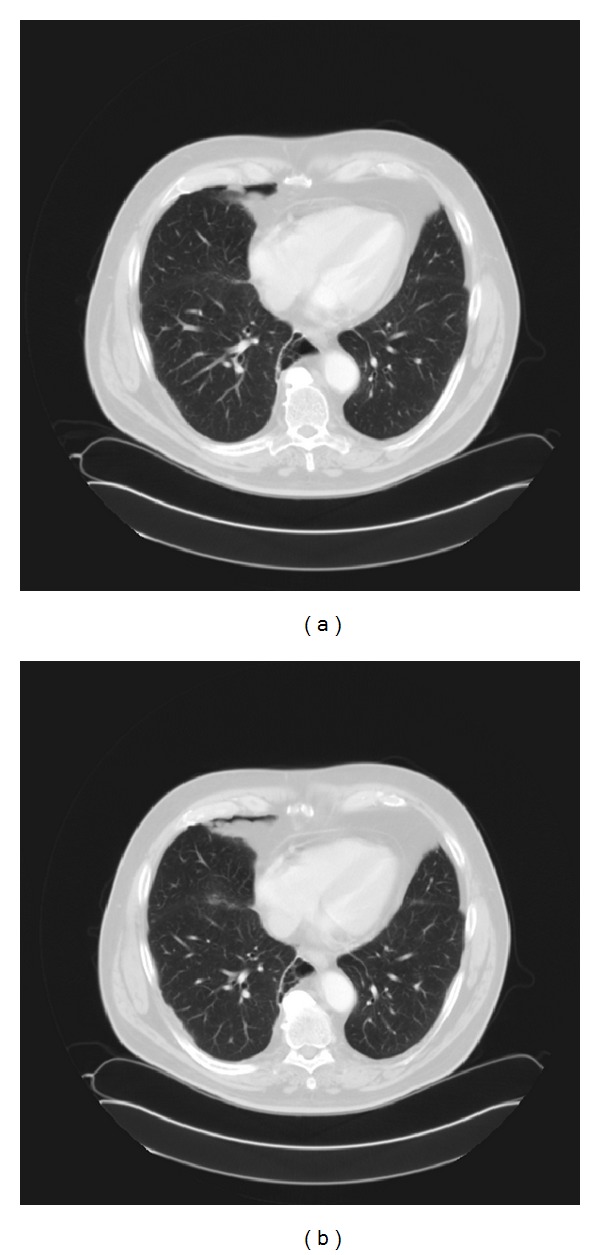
Chest CT scan in September 2007 showing a small anterior right pneumothorax.
